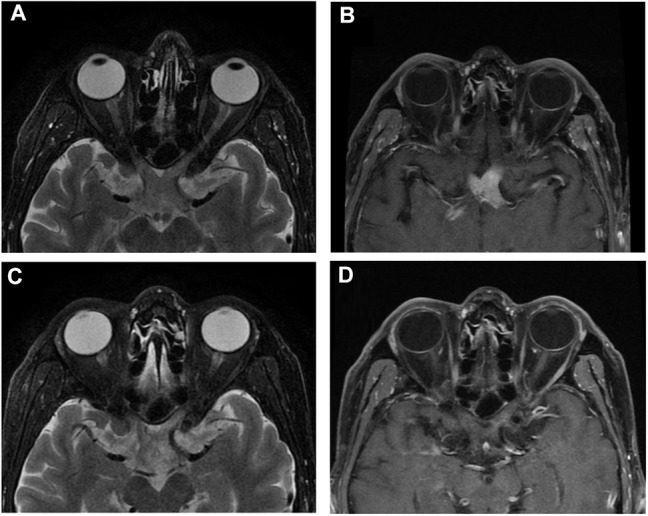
Orbital MRI of Case 1. (A,B) Orbital MRI showed chiasm enlargement in T2-weight imaging and its intrinsic homogenous enhancement of the optic chiasm in T1-weight enhanced imaging when the patient first visited the clinic. (C,D) MRI showed moderate enlargement in T2-weight imaging with a light intrinsic enhancement in T1-weight imaging of the optic chiasm after steroid treatment.
